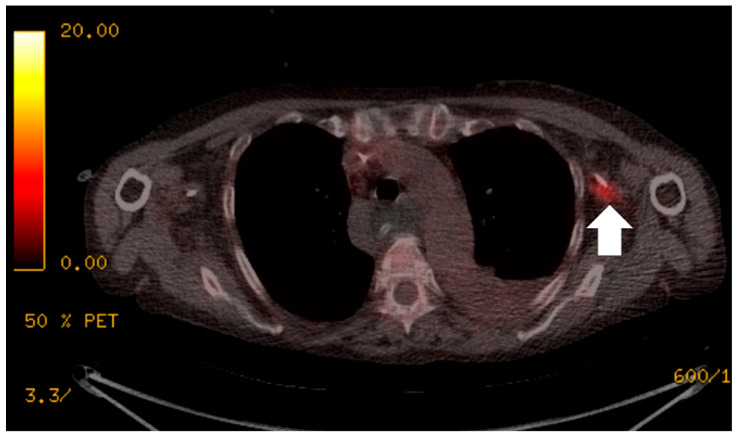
The patient presented with an elevated white cell count and raised C-reactive protein (CRP) of greater than 250 mg/L, but multiple previous image-guided drains, aspirations, line and blood cultures were negative. ^18^F-FDG PET/CT localised the infective focus to the left peripherally inserted central catheter (PICC) line (white arrow). The PICC line was removed and there was a subsequent improvement in the patient’s clinical condition.
